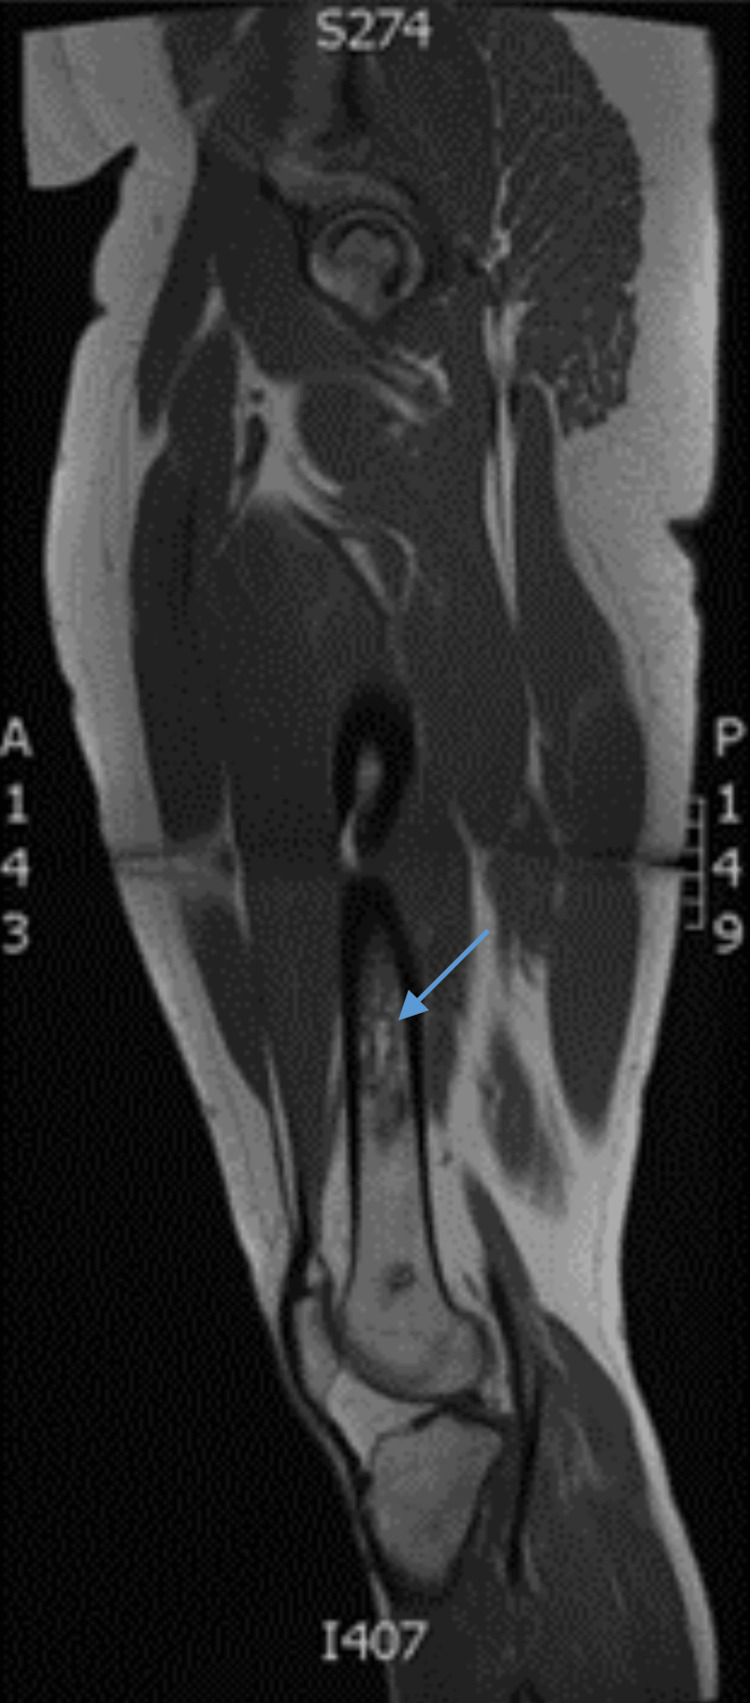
A saggital 1.5 Tesla MRI, T1 turbo-spin echo demonstrated osteonecrosis of the left femoral head occupying approximately 40% of the weight-bearing surface and an elongated bone infarct within the left mid-femoral diaphysis (blue arrow). Additional smaller bone infarcts are visible at the distal femur.
